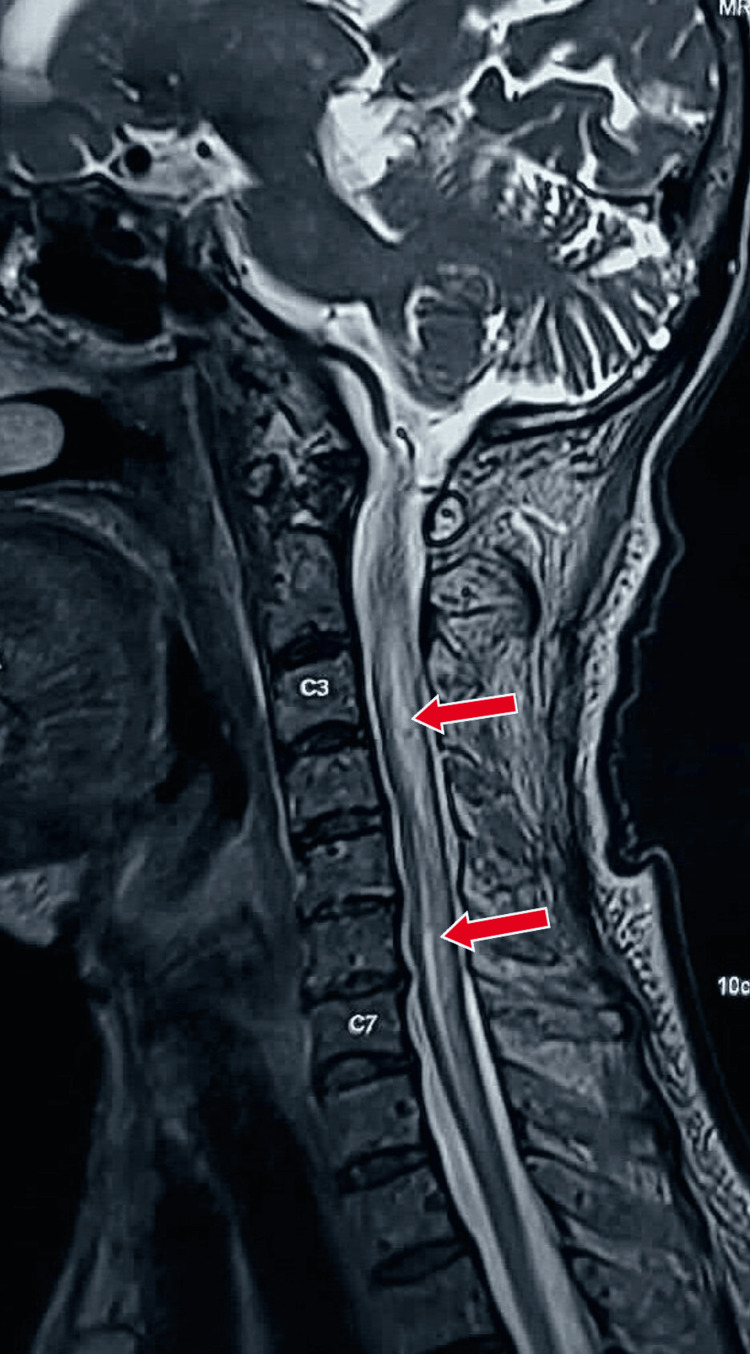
MRI cervical spine (T2 sagittal section) showing longitudinal extensive lesion of spinal cord at C2-D1 level with central cord prominence marked with red arrows. MRI: magnetic resonance imaging.
